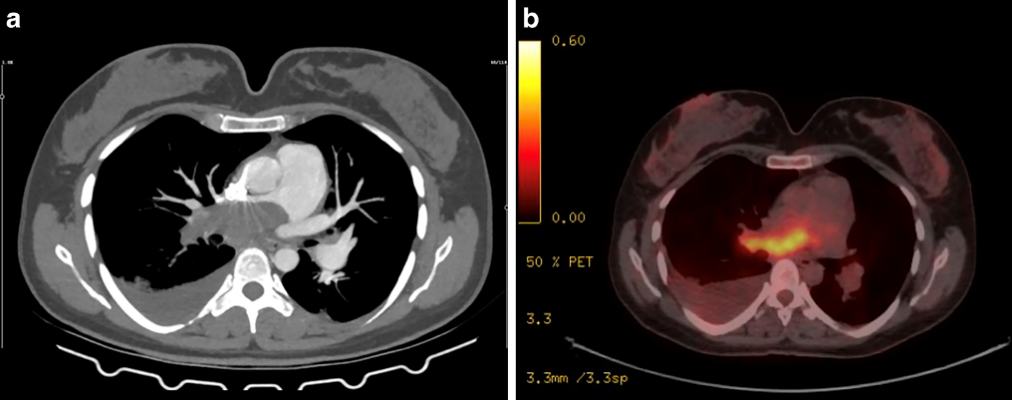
Axial computed tomographic (CT) pulmonary angiogram and 18F-fluorodeoxyglucose positron emission CT (FDG PET-CT) scans at the initial presentation. a CT pulmonary angiogram shows a large filling defect in the right main pulmonary artery extending to the subsegmental branches. b FDG PET-CT shows high metabolic activity in the right pulmonary artery consistent with CT pulmonary angiogram findings
